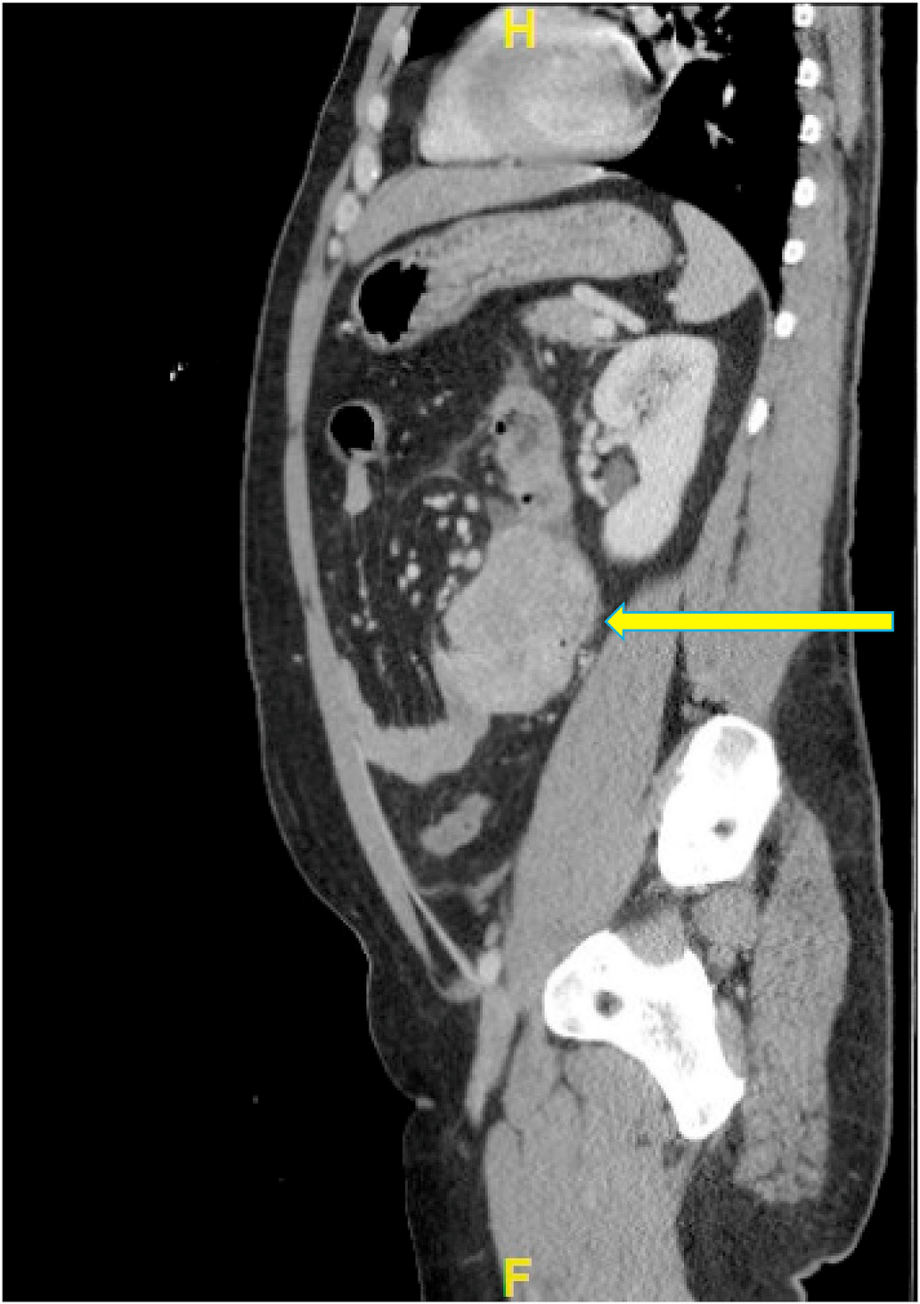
Sagittal view of abdomen/pelvis CT scan showing the GIST tumor – soft tissue mass seen in mid-abdomen. Arrow pointing to mass.
